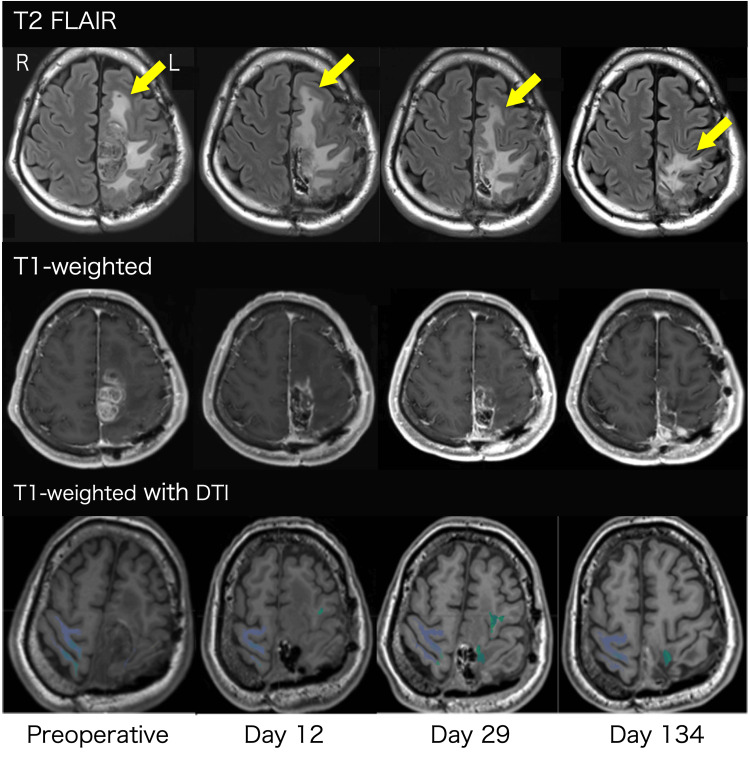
MRI T2 FLAIR high-intensity areas (yellow arrow), tumor regions in T1-weighted images, and fractional anisotropy (FA) of the corticospinal tract (CST) Preoperative evaluation was conducted 25 days before surgery. The colored area on T1-weighted images with diffusion tensor imaging (DTI) indicates the CST. FLAIR: fluid-attenuated inversion recovery
